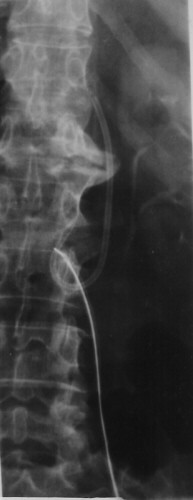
Chest X-ray showing the port in correct position while the catheter is not shown here. The catheter had moved in the right atrium, from where it was removed fluoroscopically.
